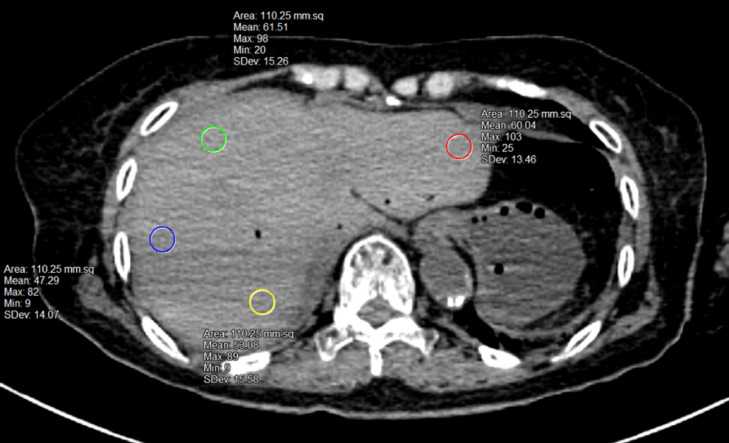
Four-point region-of-interest placement on postmortem computed tomography images using a thin-client viewer.
